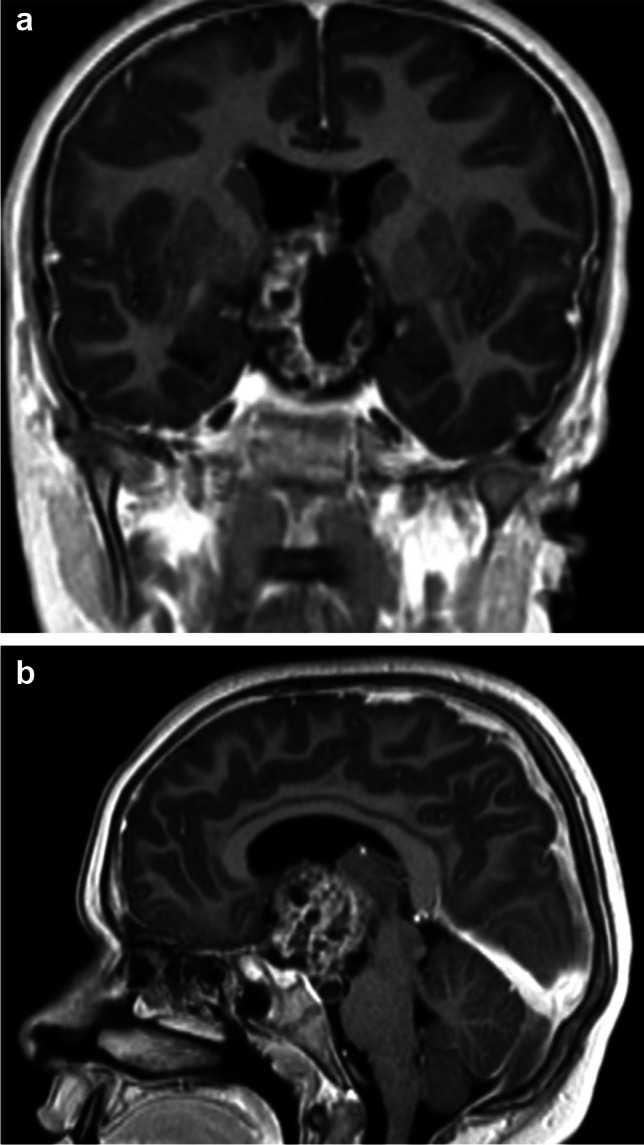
a and b Pre-operative MRI of a mixed form of craniopharyngioma before the surgical treatment
